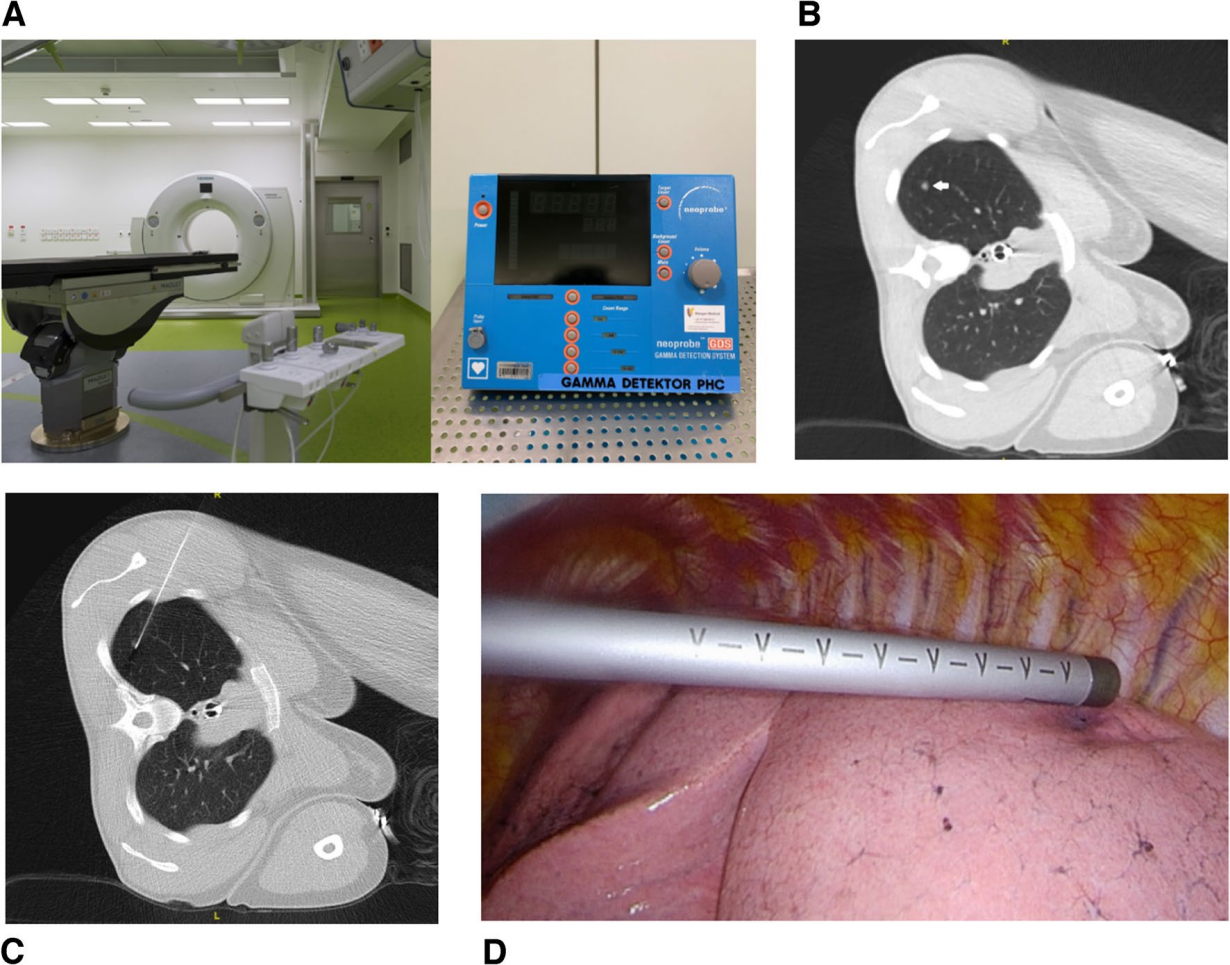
Nodule detection. A Hybrid operating room and Neoprobe console; B Planning CT scan of a 28-year-old patient with a history of clear cell sarcoma and suspected, then subsequently confirmed metastasis; C CT-guided puncture and nodule marking; D Intraoperative detection with the Neoprobe^®^ gamma detection system
